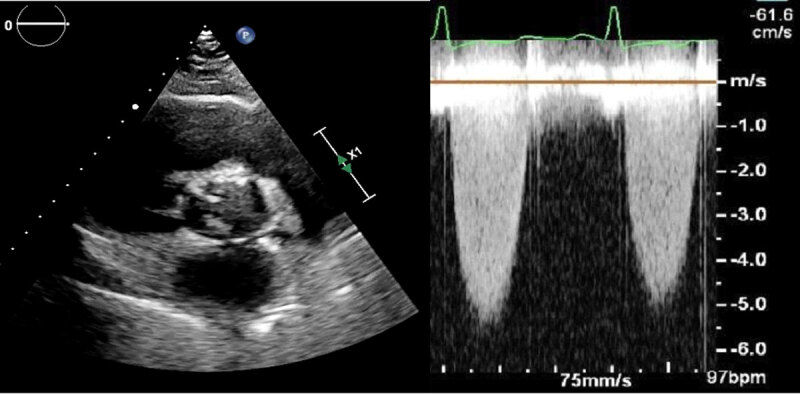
Echocardiogram of short axis view showing a bicuspid valve with increased velocity across the valve; see also at https://youtu.be/4C2kncWIOqM.
